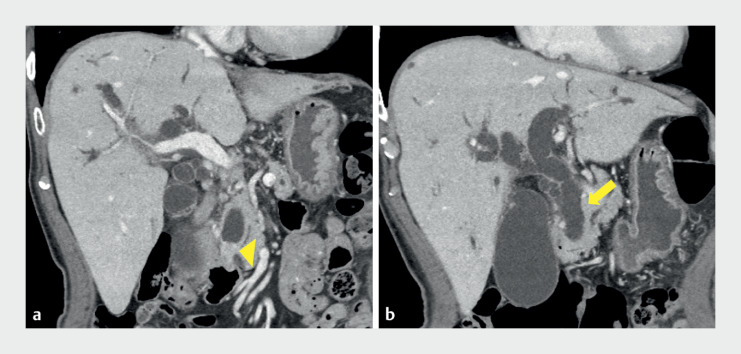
Computed tomography (CT) images on admission of an 82-year-old man with obstructive jaundice revealed:
